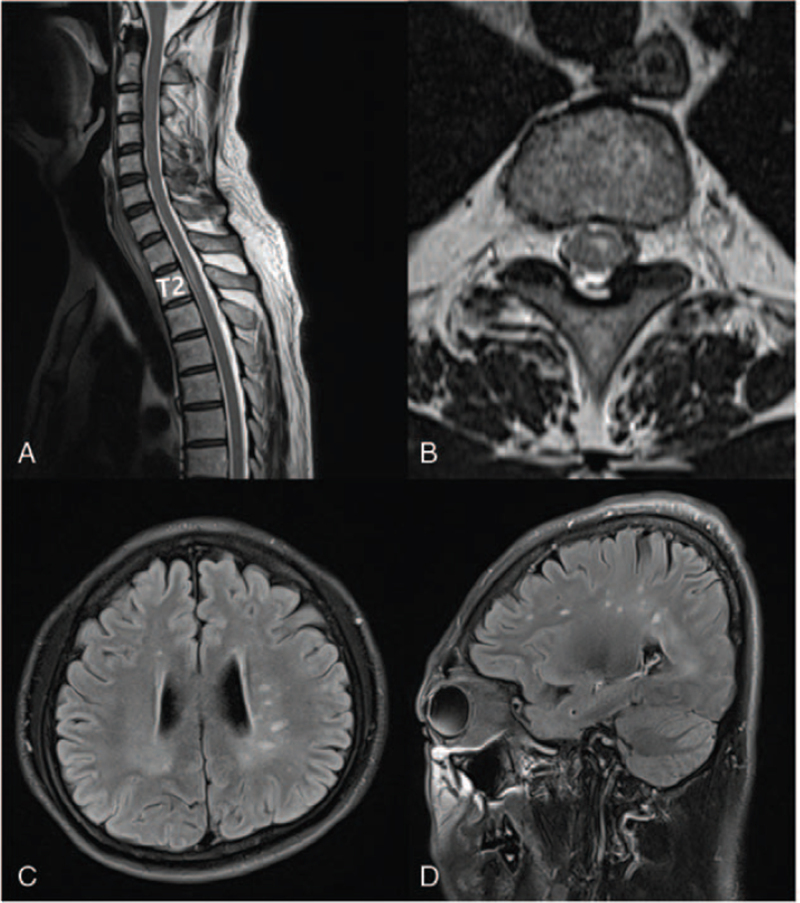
Spine and brain MRI findings during the second attack. (A, B) Sagittal and axial T2-weighted images showed intramedullary high-signal intensity lesion of the spinal cord at the level of the second thoracic vertebra. (C, D) Axial and sagittal fluid-attenuated inversion recovery images showed several high-signal intensity lesions in the periventricular and juxtacortical regions. The number of lesions had increased compared to the previous brain MRI findings. MRI = magnetic resonance imaging.
